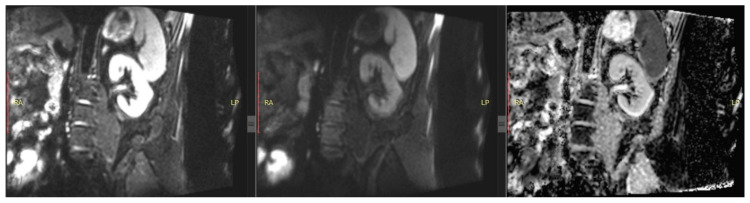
Example of kidney in DWI (b = 0 s/mm^2^, b = 400 s/mm^2^—two images from the left) and ADC map (on the right).
